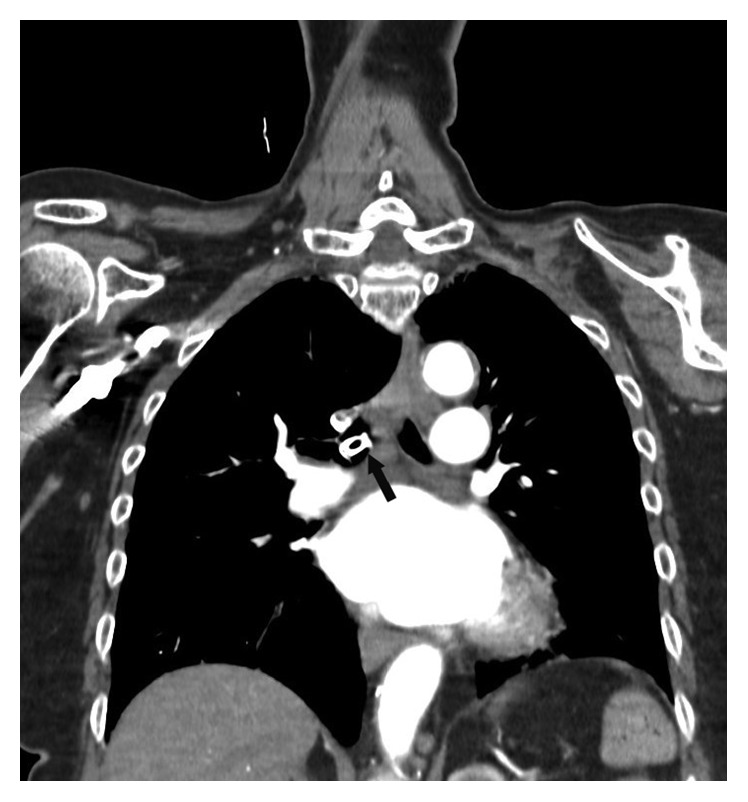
Coronal CT scan demonstrating aspirated TEP in the right mainstem bronchus.
